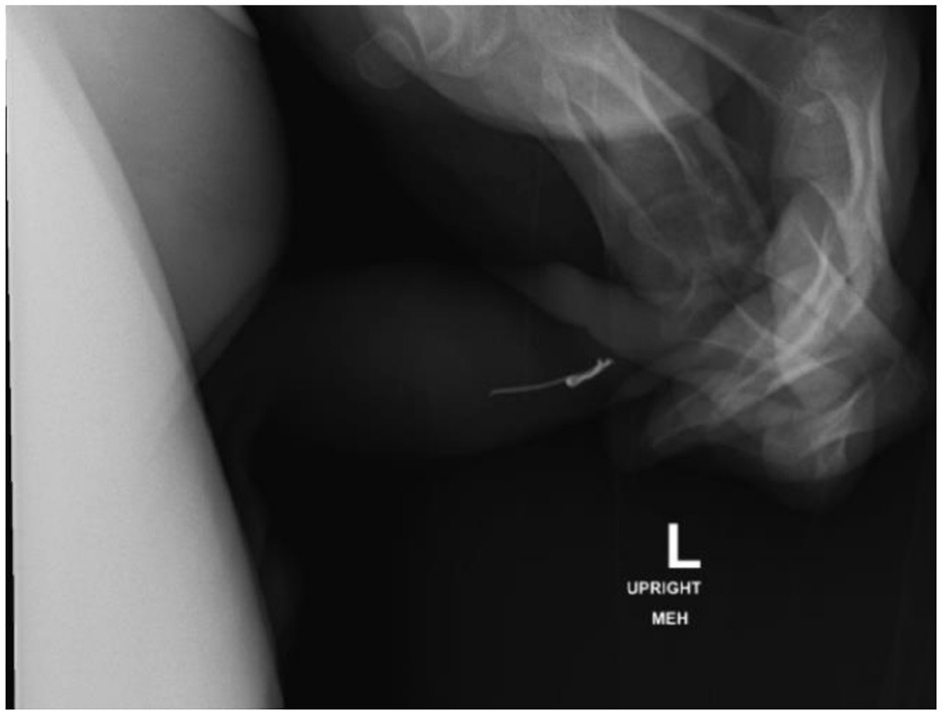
Lateral view pelvic X-ray identifies metal wire from hospital face mask in distal penile urethra, which was voided spontaneously.
